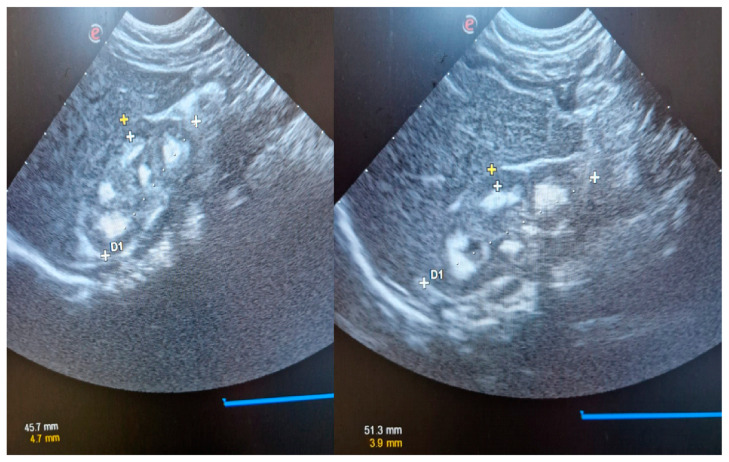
Ultrasound findings in cases two and three, revealing a bilateral hyperechogenicity of the renal pyramids.
